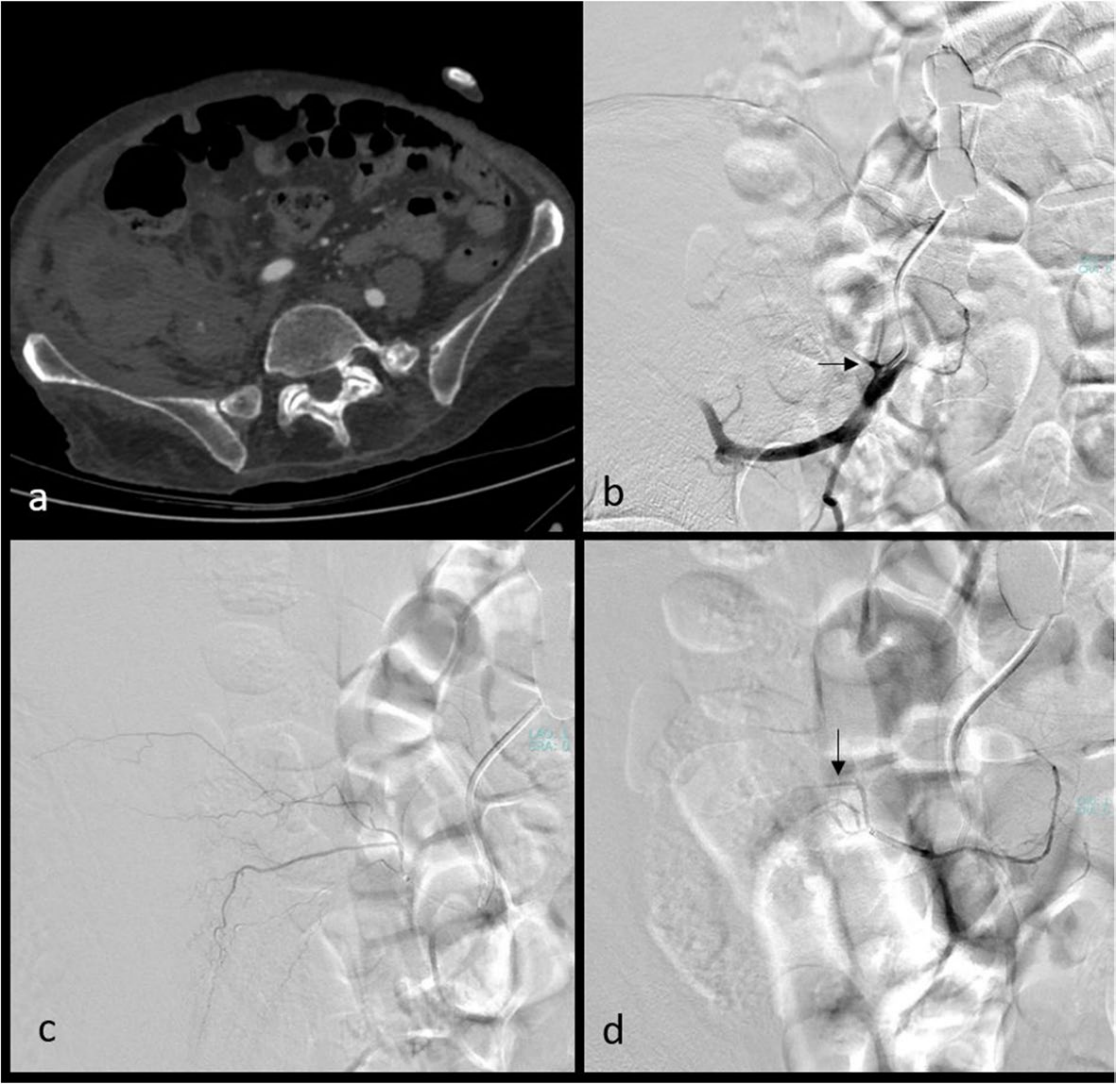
Radiological images of a 58-year-old-male patient with a right iliopsoas hematoma. Computed tomography image (a). The right internal iliac arteriogram revealed the iliolumbar artery ostium (black arrow), which was accessed via a coaxial microcatheter (b). Despite the absence of active bleeding (c), empirical embolization was performed using polyvinyl alcohol particles (250–350 microns) due to the patient’s clinical condition, and complete stagnation of iliolumbar artery flow was achieved (black arrow) (d)
